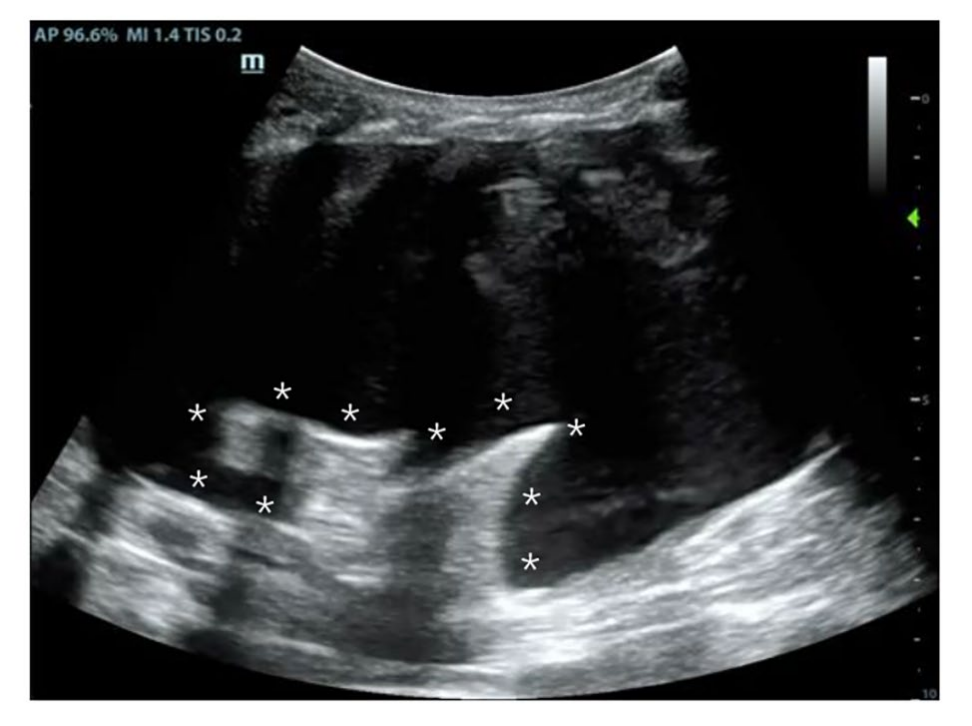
Ultrasound suggested massive pleural effusion, and severe collapsed left lung (*) with “jellyfish” sign
